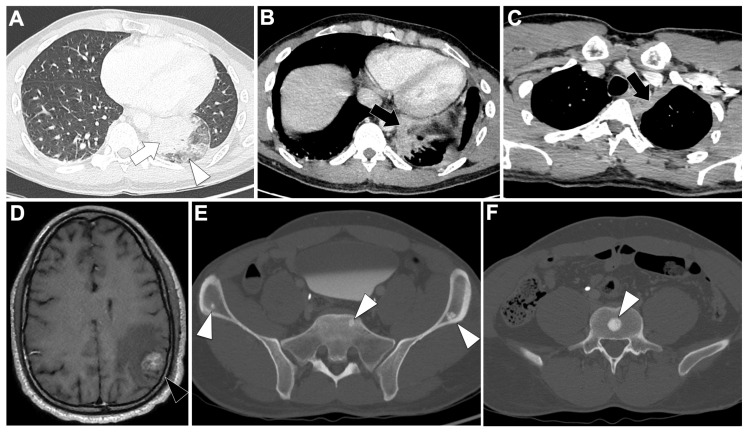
Representative baseline CT imaging findings in patients with ROS1-altered lung adenocarcinoma (LUAD). A 38-year-old man with no smoking history presented with a left lower lobe mass (white arrow) surrounded by ground-glass opacities (white arrowhead) on chest CT (A). On the mediastinal window, ipsilateral pleural soft-tissue infiltration was observed (black arrows) (B,C). Contrast-enhanced T1-weighted brain MRI demonstrated a heterogeneous parietal cortical metastasis (black arrowhead) (D). Bone-window CT images revealed multiple sclerotic bone metastases (white arrowheads) (E,F).
